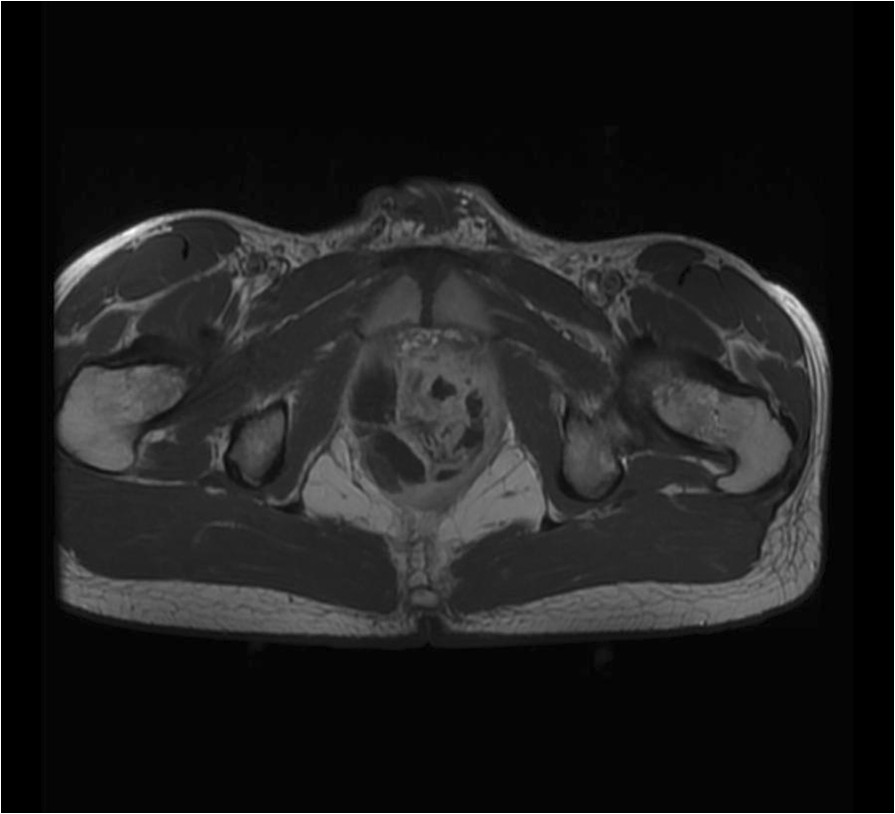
Pelvic MRI at D0. Pelvic MRI at D0 showing multiple intra-prostatic abscesses: the biggest ones are situated in the right lobe.
